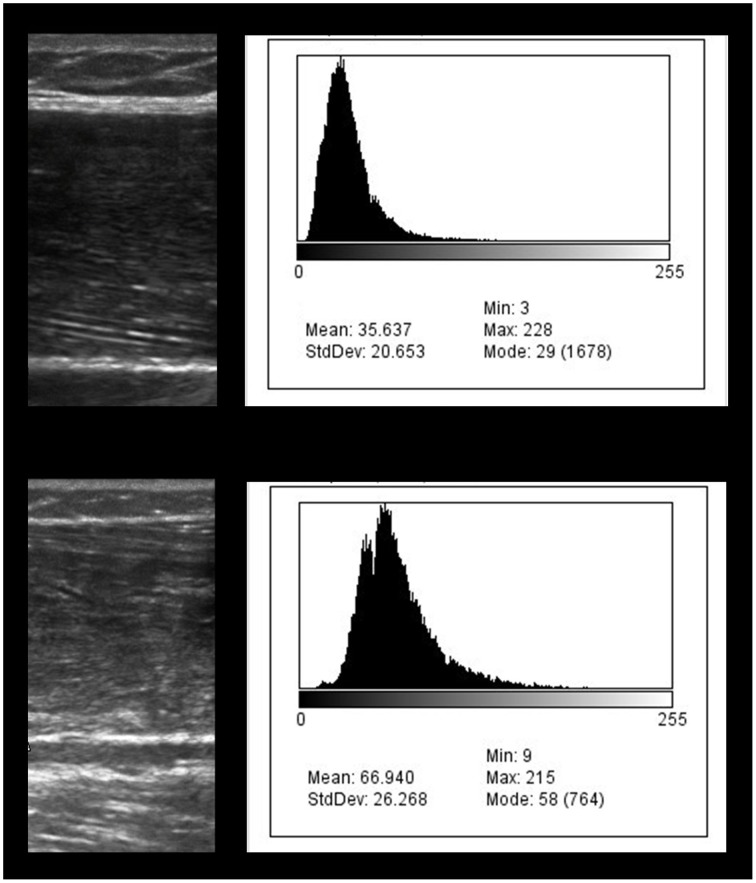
Diagnostic ultrasound image of the rectus femoris region of interest and the corresponding grayscale histogram analysis values. The exemplar images depict the diagnostic ultrasound transverse muscle images on the left and the grayscale histograms on the right. The bottom ultrasound image shows greater hyperechoic properties in comparison to the top image. The comparatively hyperechoic image characteristics of the bottom image correspond to grayscale histogram data with a wider distribution and a shift to the right which is associated with larger grayscale values. The grayscale value of the bottom image is 66.9 and may indicate a greater proportion of intramuscular adipose tissue in comparison to the top image (grayscale value, 35.6).
